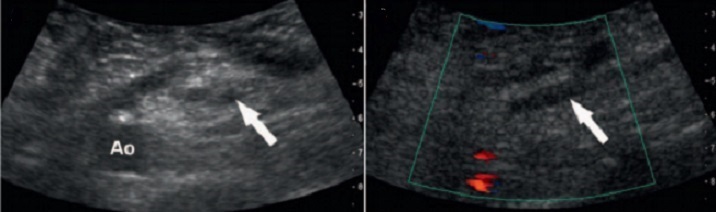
Thrombosis of the right ovarian vein showed on the doppler ultrasonography
